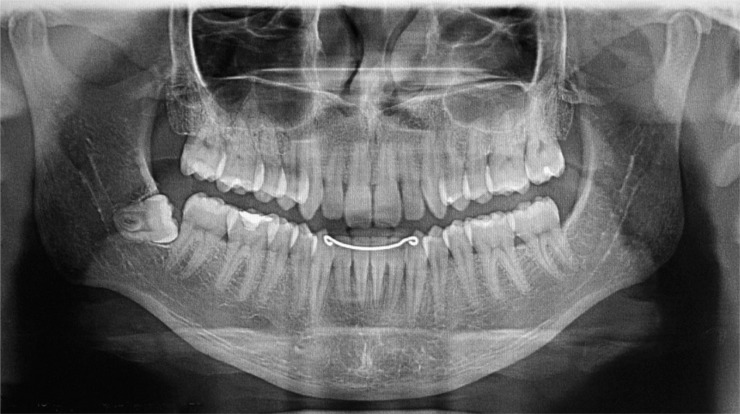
Control panoramic radiograph 10 years after treatment completion
